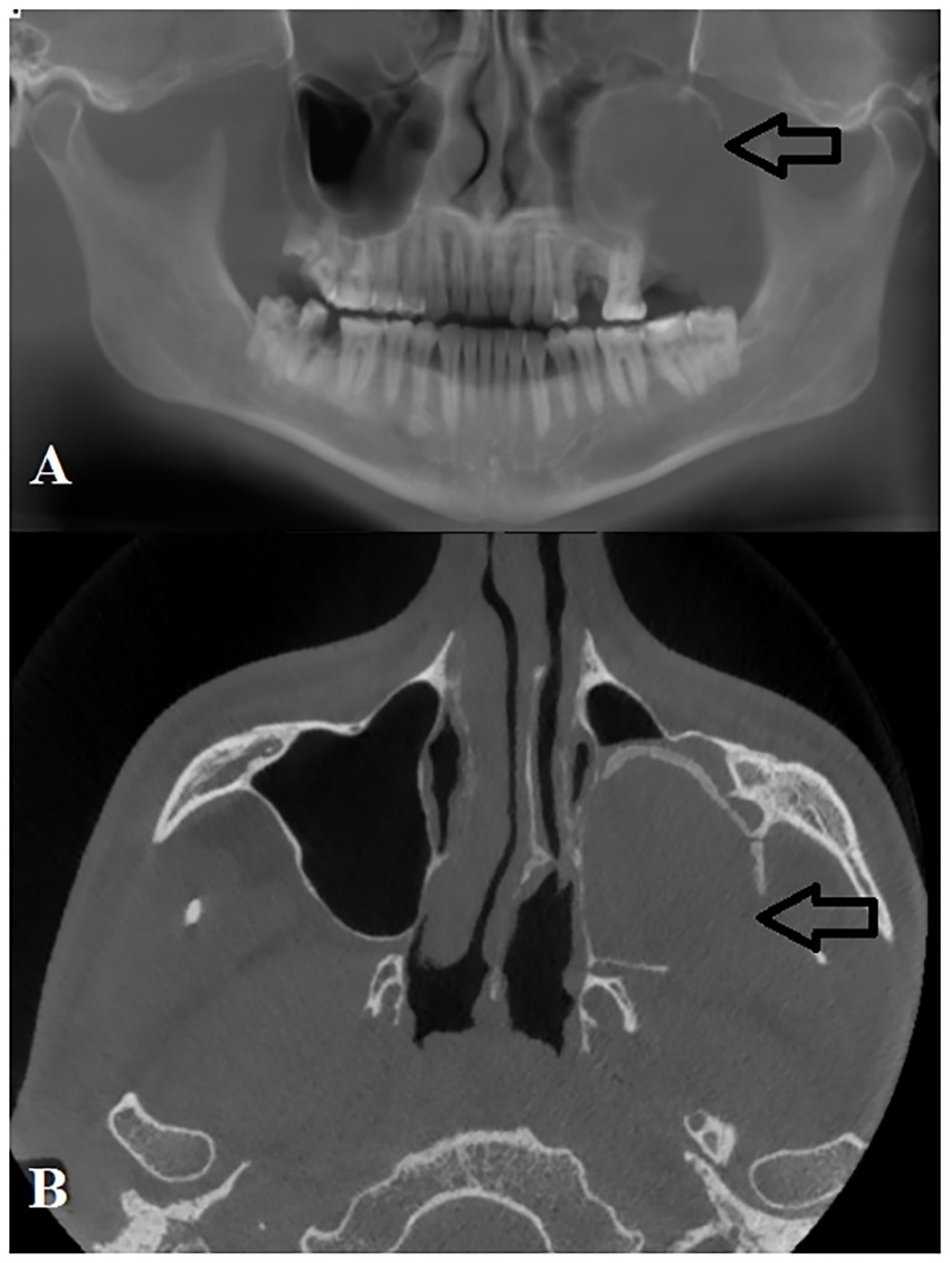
A: Panoramic reconstruction of cone beam computed tomography (CBCT) images of large residual cyst in the left maxilla that recurred following enucleation due to extension into maxillary antrum. B: Axial view of same patient showing residual cyst occupying the entire left maxillary antrum.
